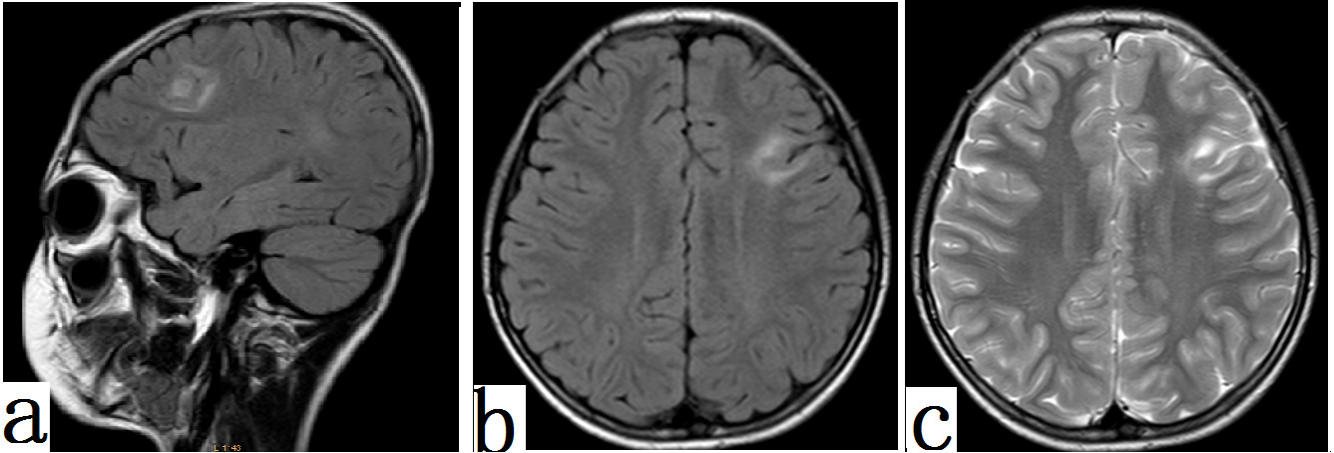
MRI features in FCD II patients Typical MRI features of focal cortical dysplasia type IIb. Diffuse thickening of the cortex in the left frontal lobe and areas of markedly increased signal could be seen on FLAIR (a, sagital; b, axial) and T2 (c,axial) (arrowhead).
